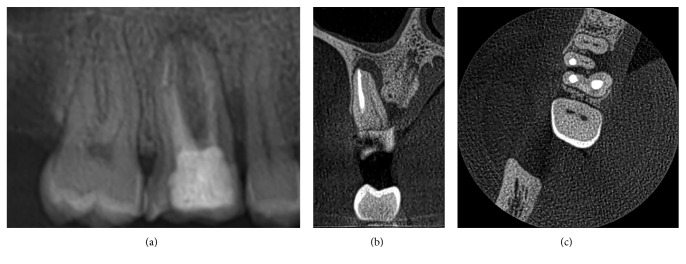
Endodontic treatment 1.6. (a) Periapical X-ray: apex endodontic treatment and periapical radiolucency. (b) CBCT  sagittal section: apex endodontic treatment MB, untreated MB2, and periapical radiolucency. (c) CBCT transversal section: untreated MB2.
